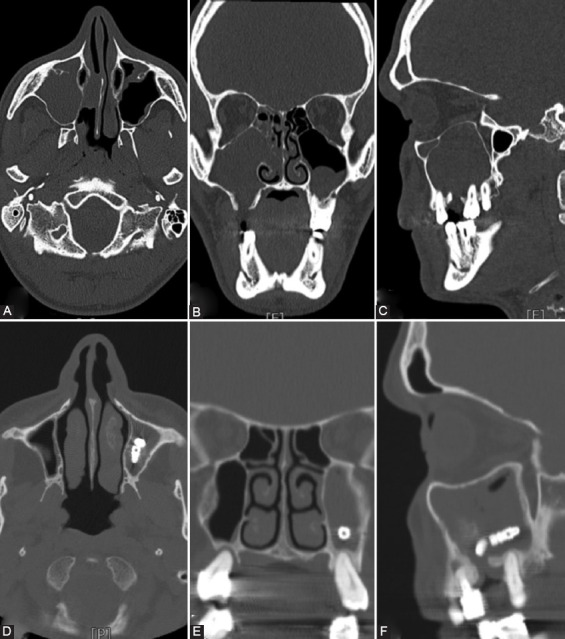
Upper panel: computed tomography scan in the axial (A), coronal (B), and sagittal (C) planes of a patient with right maxillary sinusitis and a large oroantral fistula. Lower panel: computed tomography scan in the axial (D), coronal (E), and sagittal (F) planes of a patient with a wide floor defect of the left maxillary sinus associated with implant displacement and complete sinus obliteration.
